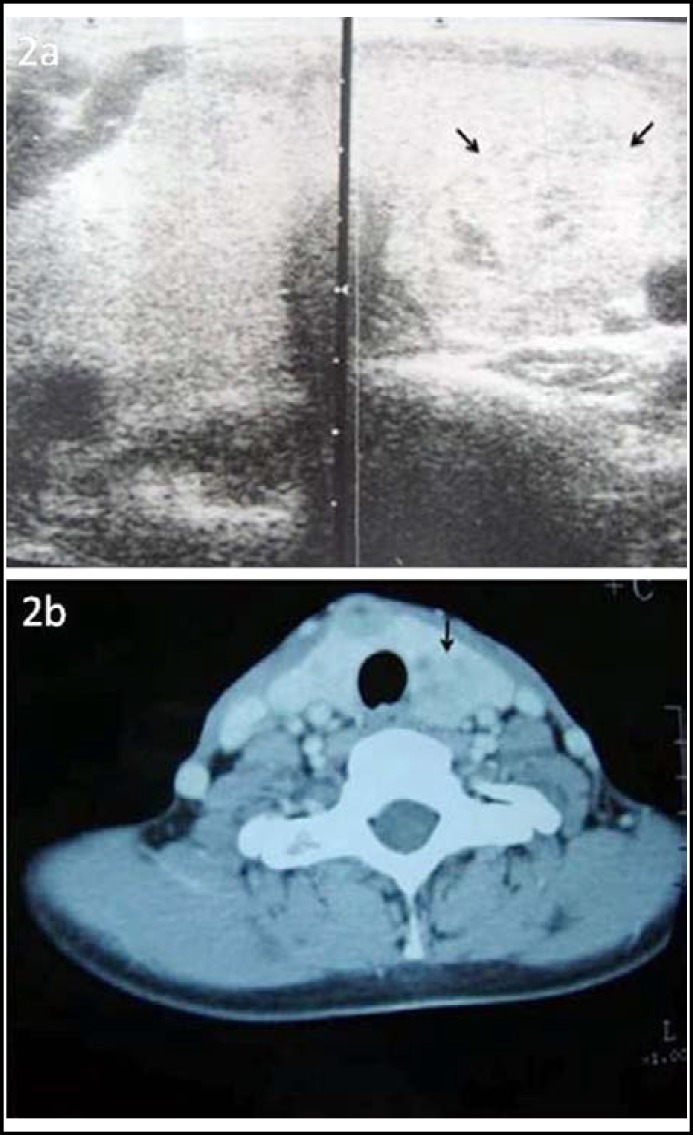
Thyroid ultrasound displaying a solitary heterogeneous nodule which is 55 X 51 mm in size. Fig.2b: Computerize tomography imaging of the neck demonstrating the same thyroid nodule.
